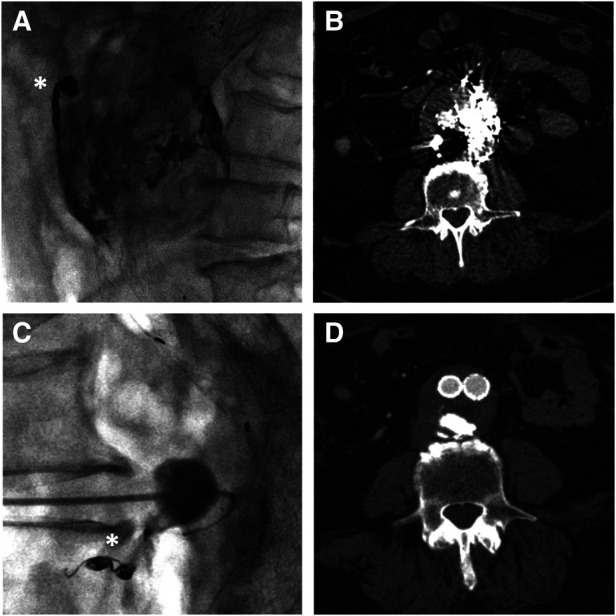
Radiopacity and strike artifacts comparison between onyx (top, figures (A,B), containing tantalum) and easyx (bottom, figures (C,D), containing iodine) on two type II endoleak embolization on fluoroscopy (A–C) and CTA follow-up (B–D). Coils in fluoroscopic images (A–C) are indicated by white asterisks.
